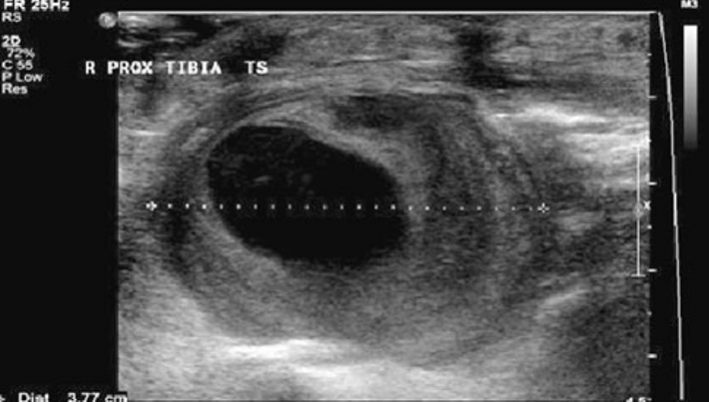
Ultrasound scan around the pin sites revealing a large mass with turbulent flow, suspicious of a pseudoaneurysm
